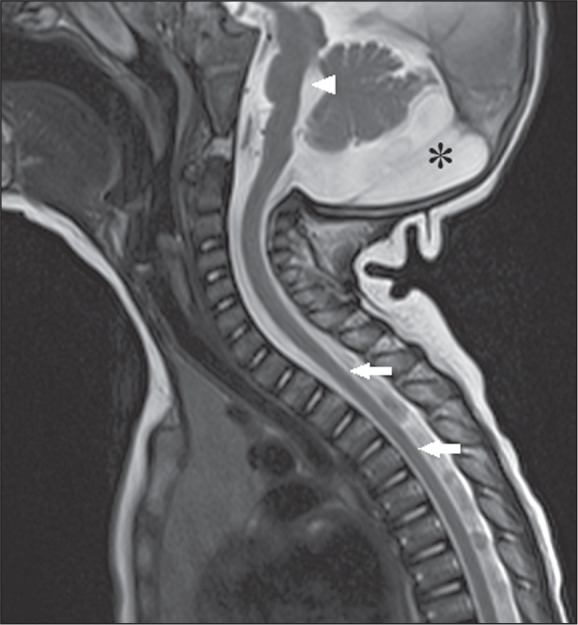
Zika. Sagittal T1-weighted MRI sequence showing narrowing of the thoracic
